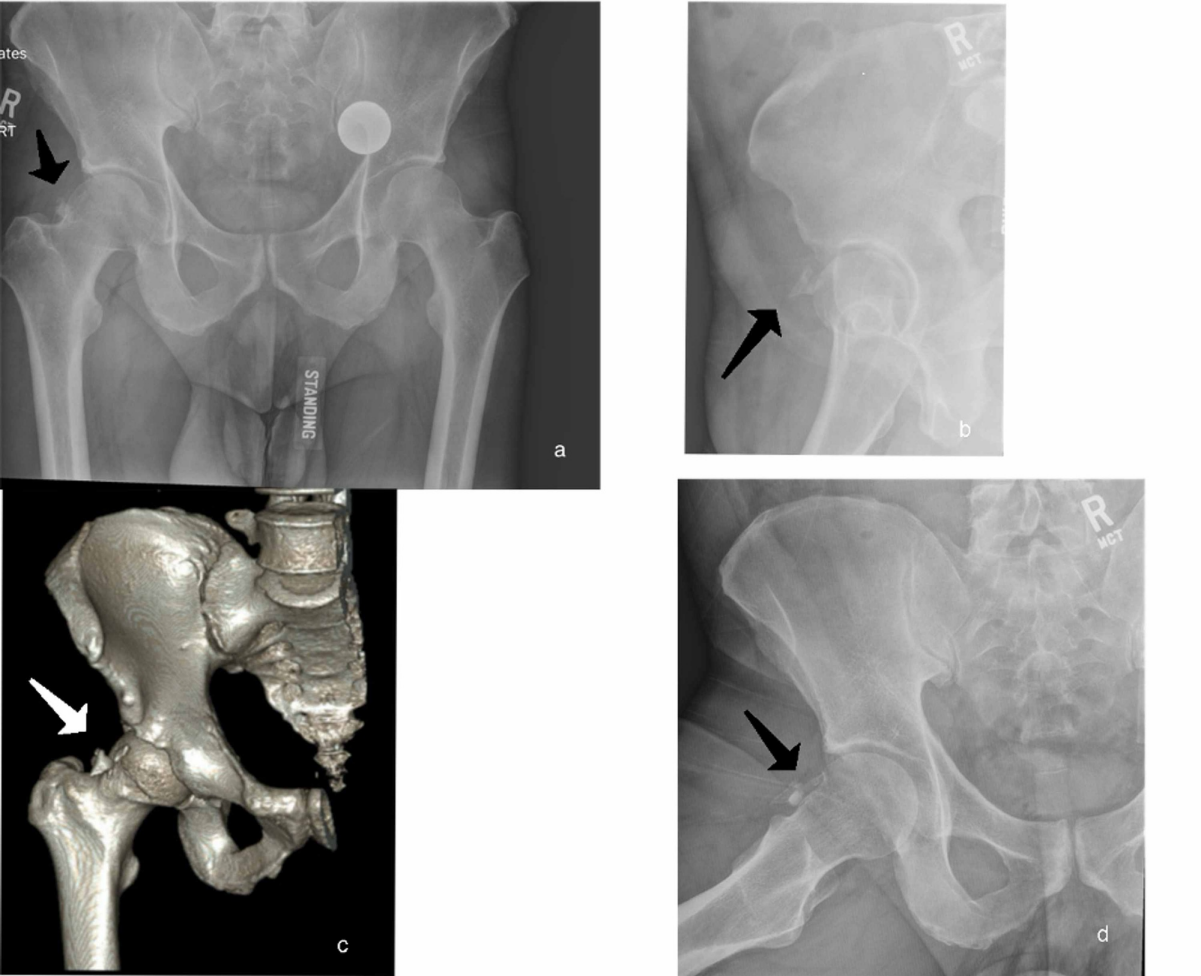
Case 2: Preoperative Imaging (a) AP pelvis with HO seen on the left hip (arrow). (b) Modified Dunn view showing grade 1 HO in the hip capsule (star). (c) False-profile view showing HO (arrow). (d) 3D reconstruction CT-image of the left hip demonstrating HO (star).
